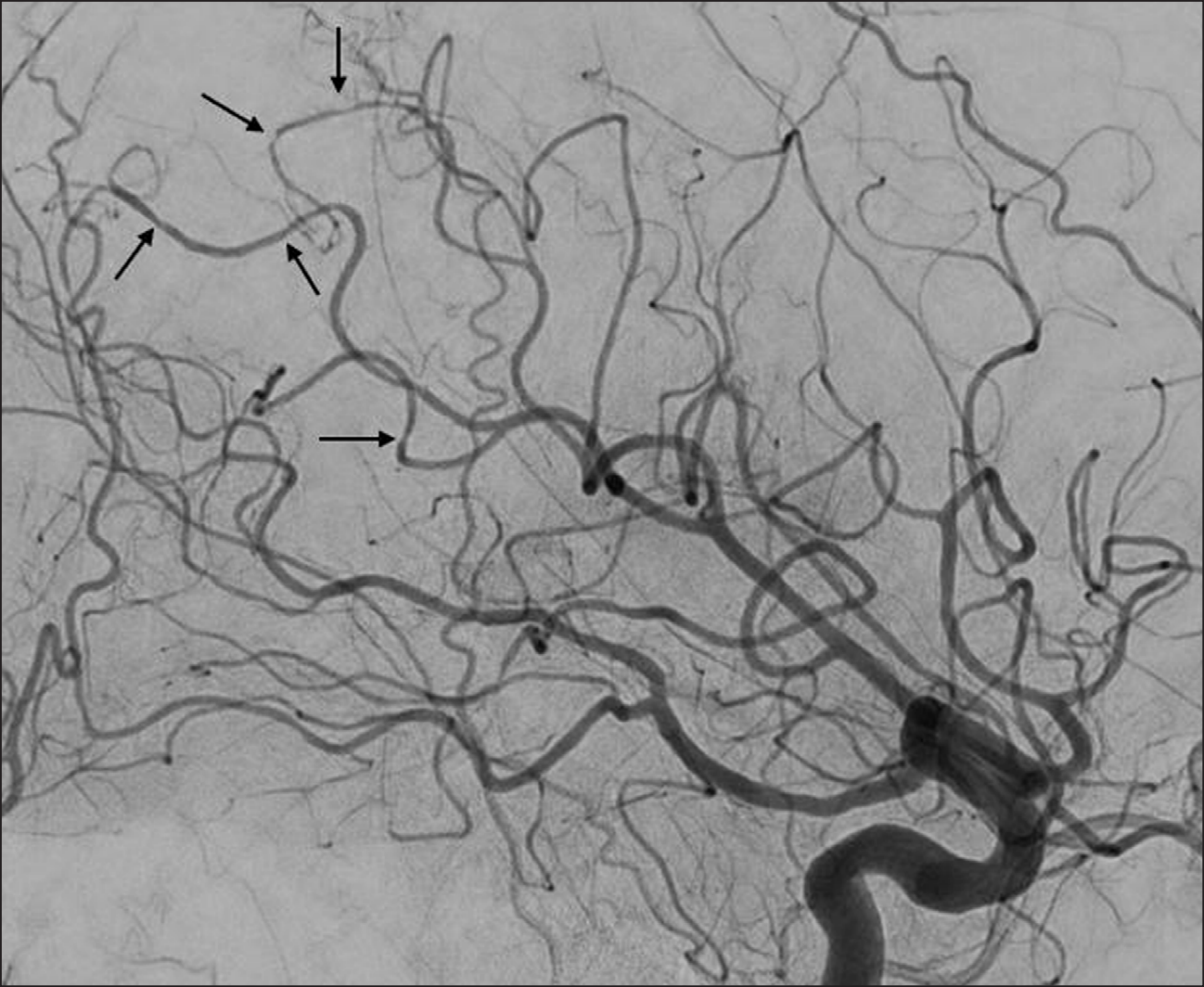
A complementary MRI investigation was performed using a black-blood sequence, which is a high-resolution 3D vessel wall magnetic resonance (VW-MR) technique done before (Figure 3A) and after (Figure 3B) intravenous injection of Gadolinium. It demonstrated multifocal arterial wall thickening and circumferential enhancement on the middle cerebral arteries (arrows on Figure 3B). The Figure 3C magnifies the left middle cerebral artery and clearly shows the thickening and the enhancement of the arterial wall with narrowing of the lumen (arrow) in opposite with the proximal part of the artery which appears normal (arrowhead).
